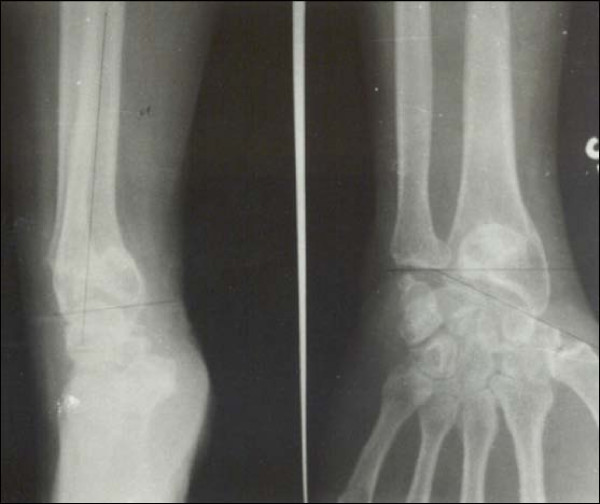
Case 2 Radiograph at 7 year followup. Anteroposterior and Lateral view.
